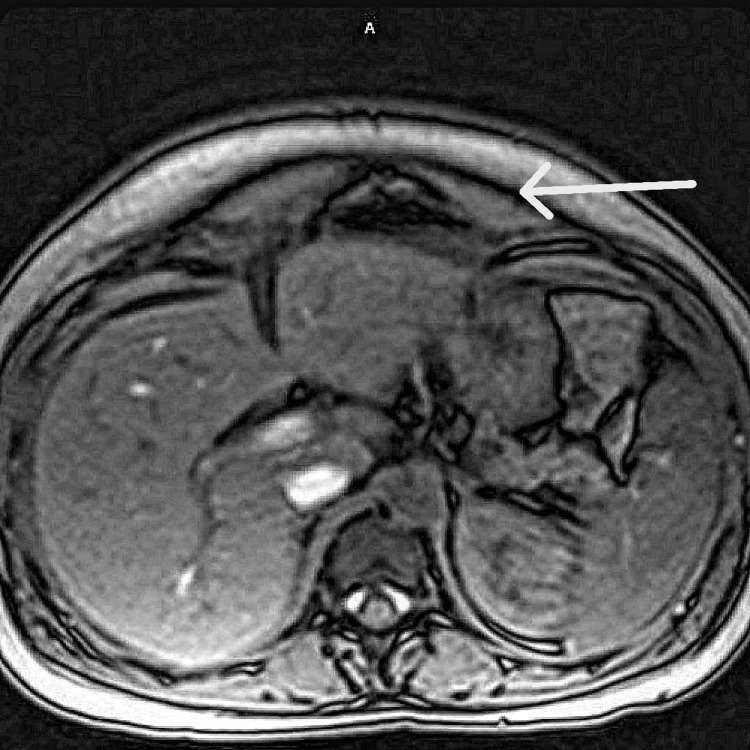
Axial T1-weighted MRI of the abdomen demonstrating possible free air at the anterior abdominal wall. Axial T1-weighted MRI of the abdomen with an arrow pointing at a focus of signal void, suggesting moderate volume intraperitoneal air in the upper central abdomen. Given the recent cesarean delivery, these findings were initially considered potentially postoperative in nature, contributing to diagnostic uncertainty.
